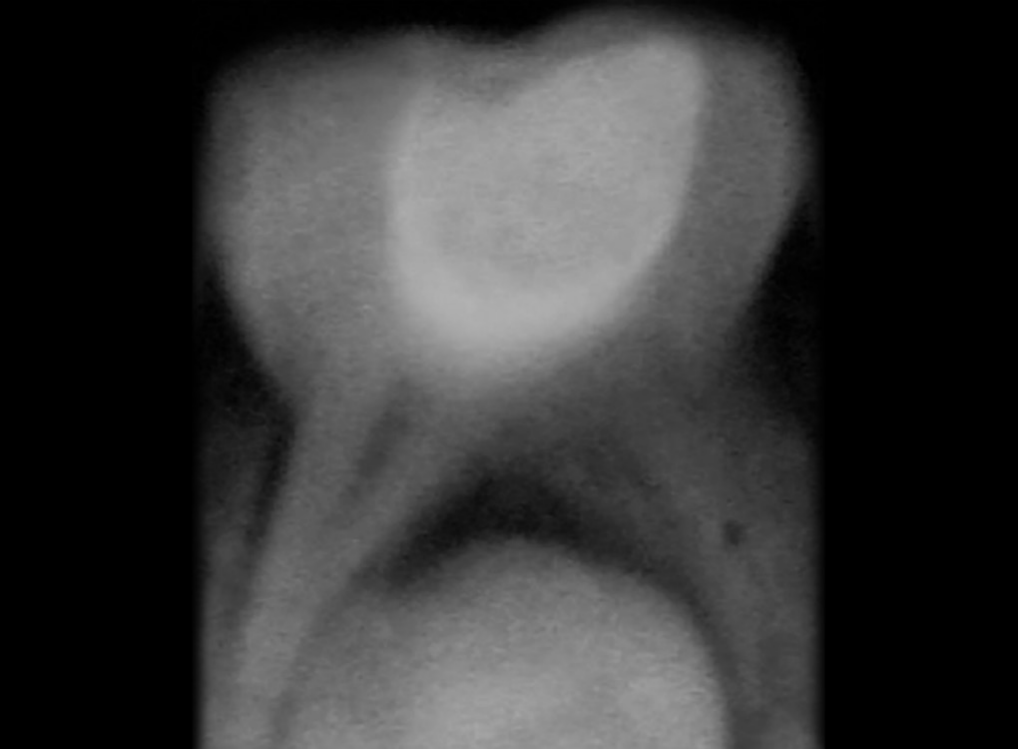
Postoperative radiograph after 6 months of 85
